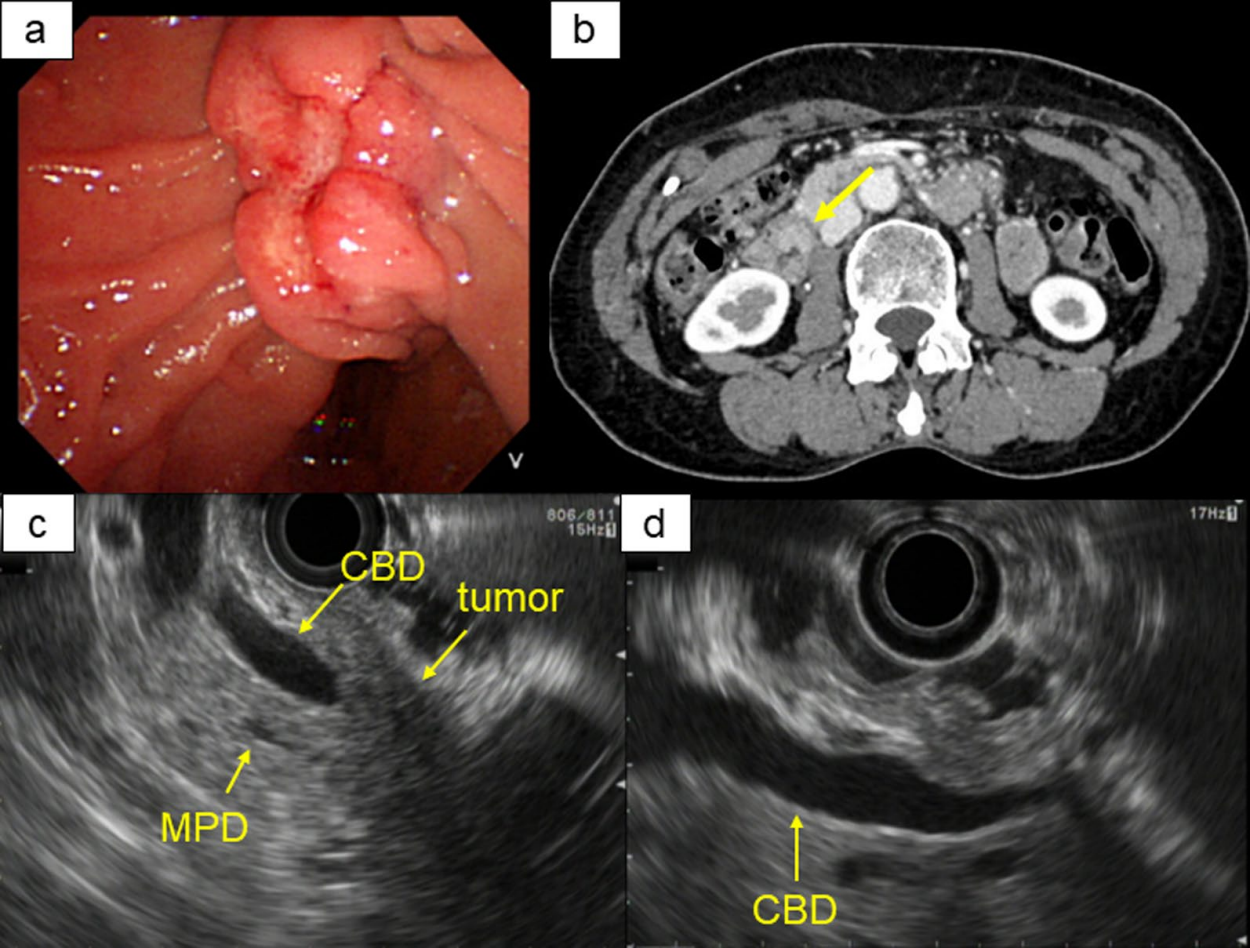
Preoperative examination. a Endoscopy revealed an ulcerative lesion in ampulla of Vater. b Contrast-enhanced CT showed a 1.5-cm enhancing nodule around papilla of Vater. c, d EUS suggested that the tumor may have invaded the pancreatic parenchyma. CT, computed tomography; EUS, endoscopic ultrasound
